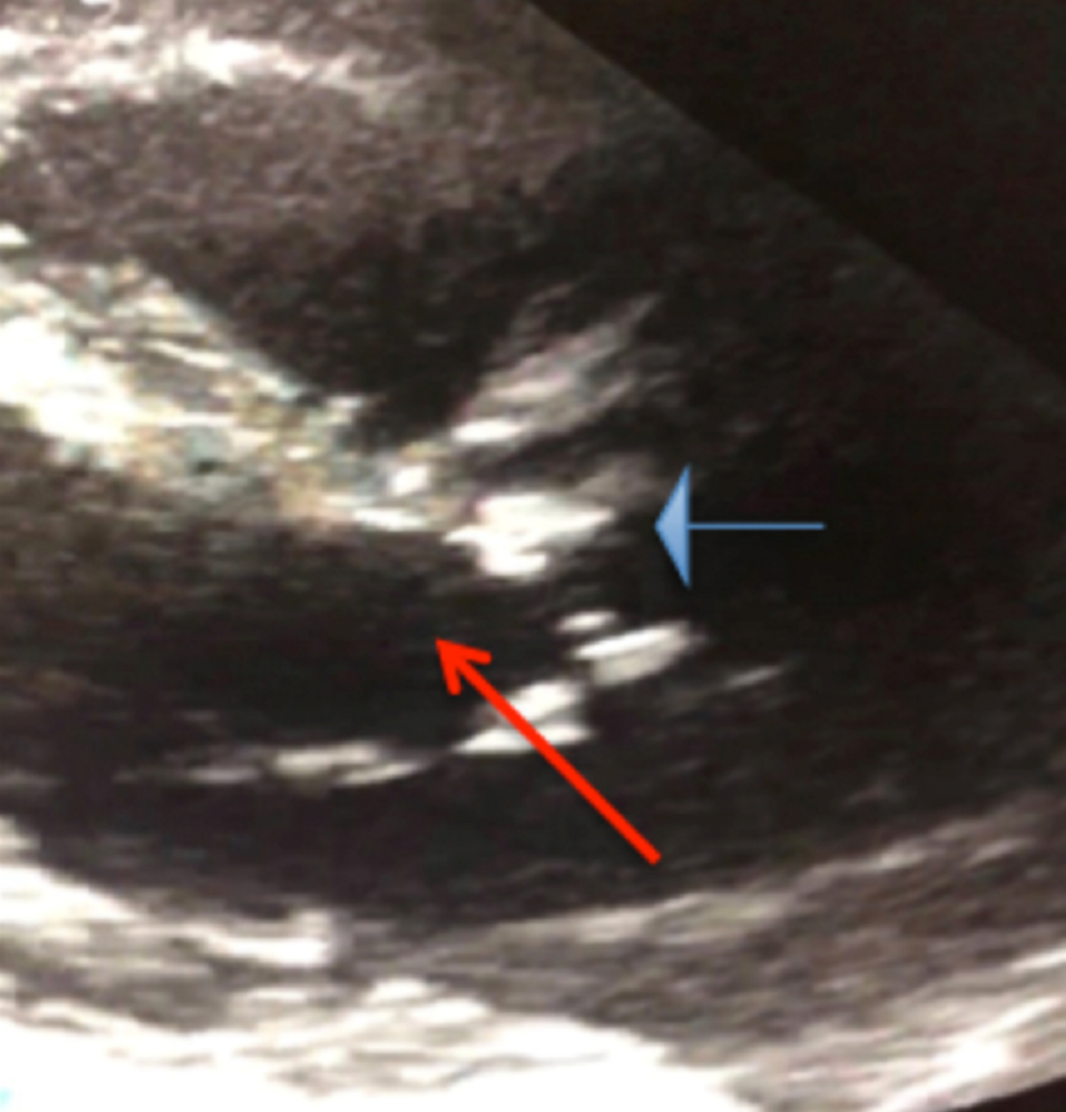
Parasternal long axis view of a two-dimensional echocardiogram, showing a calcified and stenotic aortic valve (blue arrow) and left ventricular outflow tract (red arrow).
